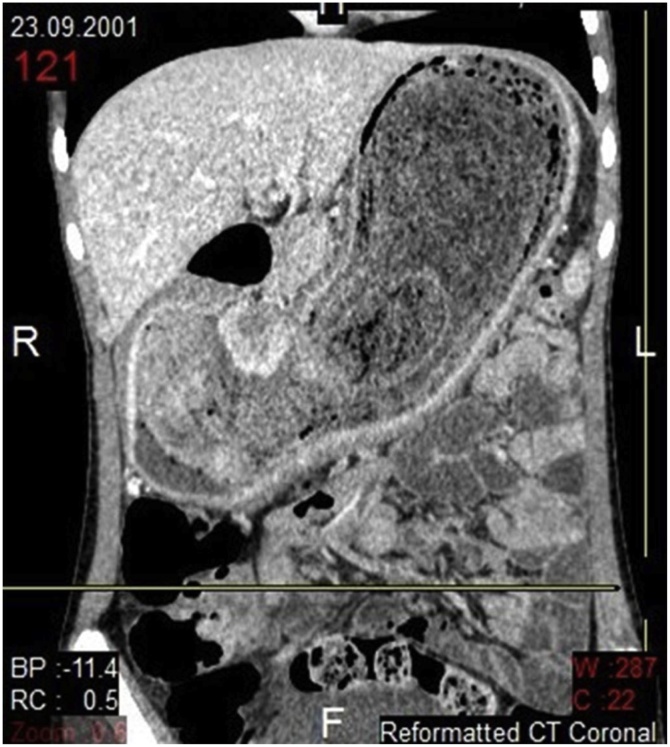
Contrast enhanced CT, Coronal view: Huge, well defined, multi-layered, heterogeneous, solid appearing, non-enhancing mass in the gastric lumen extending from the gastric fundus to the pyloric canal. Some of the layers of the mass are heterogeneously hyperdense. The mass lesion is separated from the gastric wall by gastric fluid. No evidence of abnormal gastric mural thickening.
